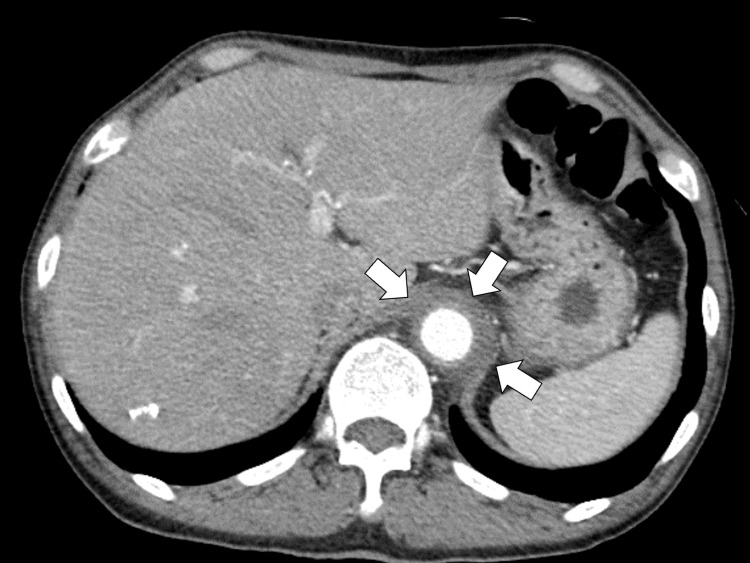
Contrast-enhanced abdominal computed tomography showing the wall thickening of the descending aorta (white arrows)
